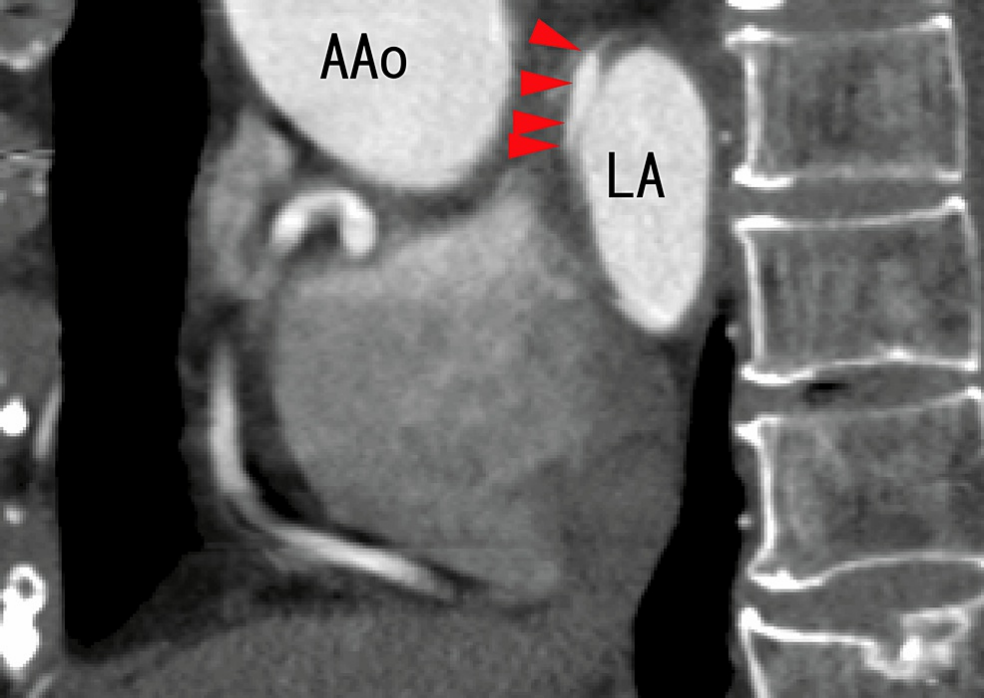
Sagittal images from an 80-MDCT scan obtained after one month of heparin and warfarin treatment The location was similar to that of the 80-MDCT scan images in Figure 5. There was a diverticulum on the anterior wall of the LA (arrowheads). The darker areas of the LA and LAD in Figure 5 became clearer, indicating that the thrombi had resolved. The distance between the anterior wall of the LAD and the anterior wall of the LA became shorter than that in Figure 5, indicating that the LAD decreased. AAo, ascending aorta; LA, left atrium; MDCT, multidetector computed tomography
